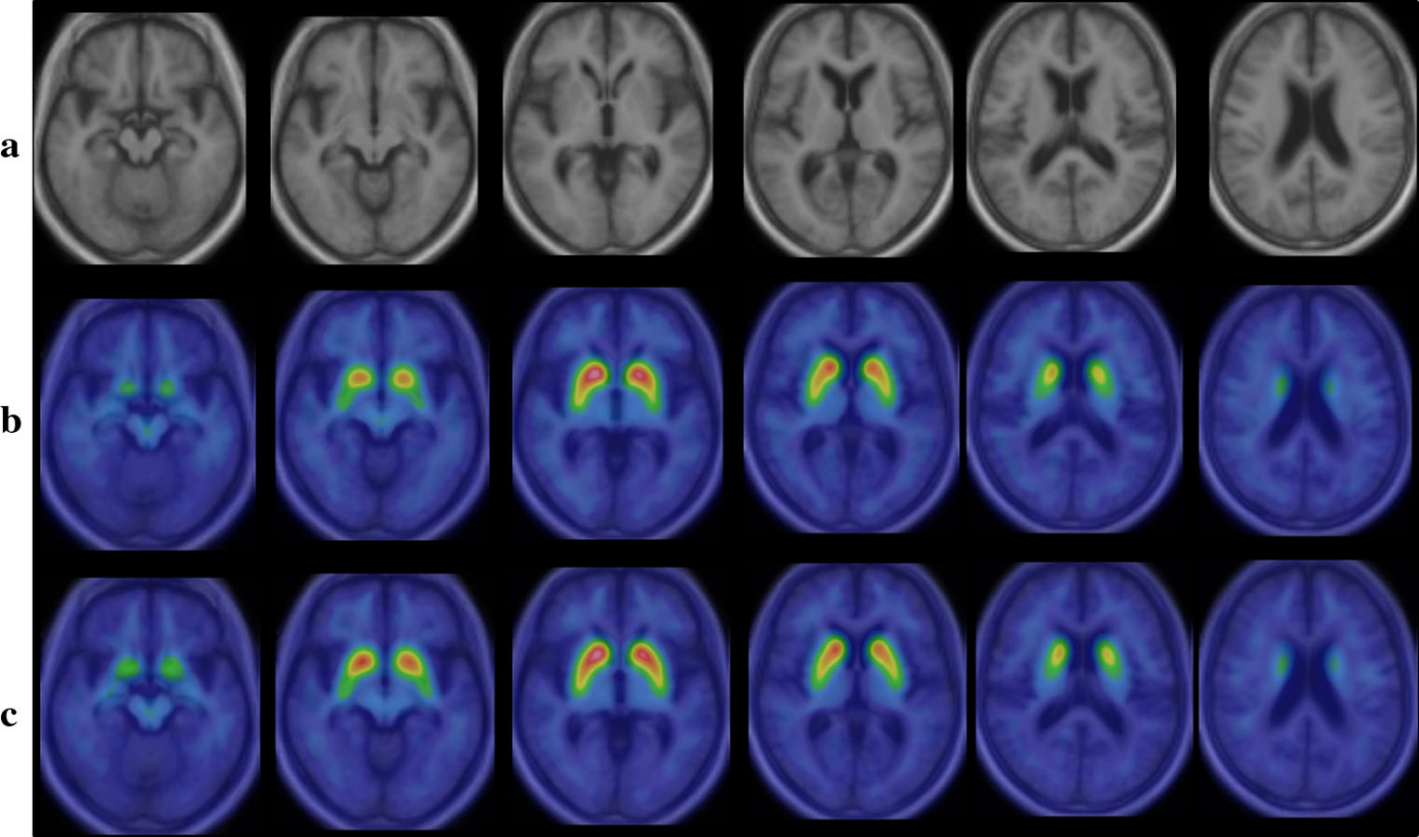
Averaged MRI images of parkinsonian patients (a), I-123 FP-CIT images normalized by the MRI-based method (b) and I-123 FP-CIT images directly normalized by the FP-CIT template-based method (c). The latter two sets of I-123 FP-CIT images are displayed superimposed on MRI images of parkinsonian patients
